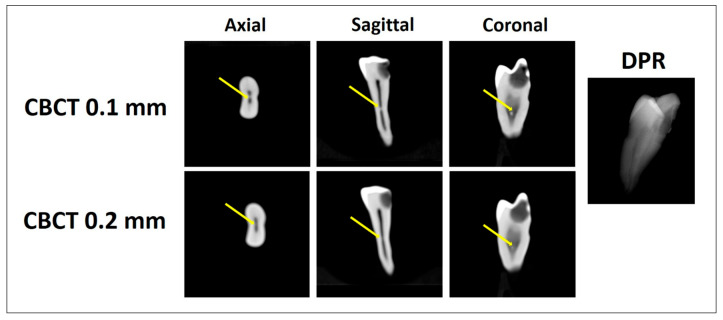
Pulp stone detection using CBCT 0.1 mm, CBCT 0.2 mm (axial, sagittal, and coronal planes), and DPR. The arrows indicate a radicular pulp stone detected by CBCT 0.1 mm and CBCT 0.2 mm but not by DPR.
